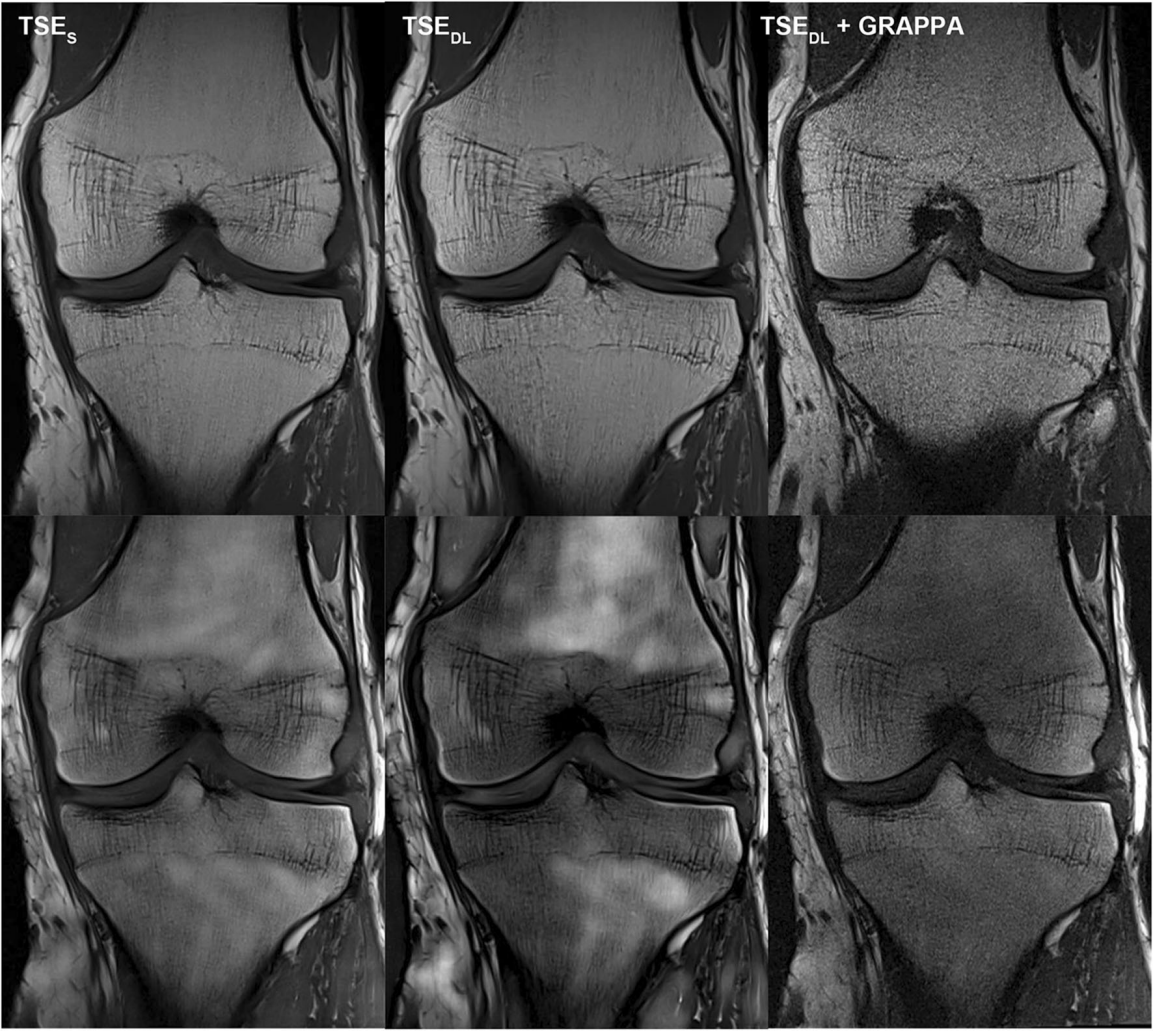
Comparison of different reconstruction techniques and SNR for standard and deep-learning T1 TSE imaging of the knee at 3 T. Exemplary visualization of different reconstruction techniques (upper row) and signal-to-noise ratio (SNR) as SNR maps (lower row) of T1-weighted TSE in coronal orientation of the knee acquired at 3 T. On the left, TSE_S_ dataset reconstructed with a standard GRAPPA reconstruction. In the middle, TSE_DL_ dataset reconstructed with the DL technique and, on the right, TSE_DL_ dataset reconstructed with a standard GRAPPA reconstruction. Compared to the TSE_S_ (upper row, left), the TSE_DL_ reconstructed with GRAPPA (upper row, right) shows higher noise levels and a decrease of SNR (lower row, left and right). The TSE_DL_ reconstructed with the DL technique (lower row, middle) shows lower noise levels and an increase of SNR compared to TSE_DL_ reconstructed with GRAPPA. TSE_S_ (left) and TSE_DL_ reconstructed with the DL technique (middle) are comparable concerning the noise levels and SNR
